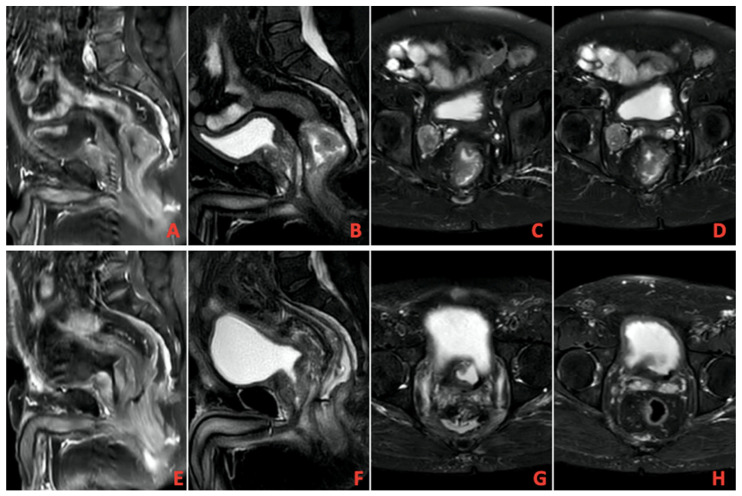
(A–D) Pretreatment imaging, (E–H) Posttreatment imaging on magnetic resonance imaging (MRI).
